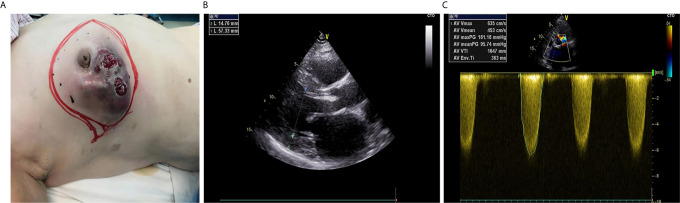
Preoperative information of the patient. (A) Appearance of the breast. (B) Two-dimensional echocardiography: the enlarged left ventricle and thickened left ventricular wall. (C) M-mode echocardiography: accelerated systolic forward flow through aortic valve, increased maximum and average aortic transvalvular pressure difference.
